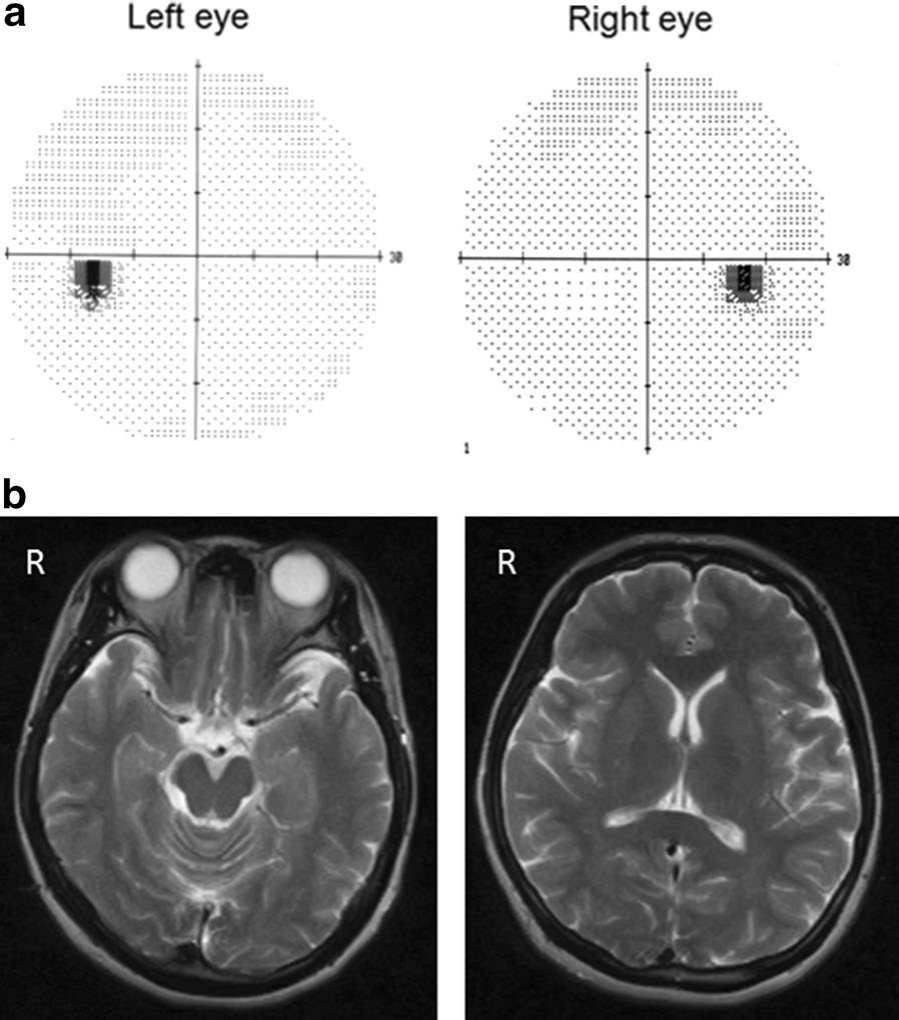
Visual field and magnetic resonance imaging after treatment. a Left homonymous hemianopsia improved at 2 days after infusion of a free-radical scavenger and anticoagulant. b MRI at 1 month after treatment. The lesions in the basal ganglions and occipital lobe were no longer visible in the follow-up MRI
